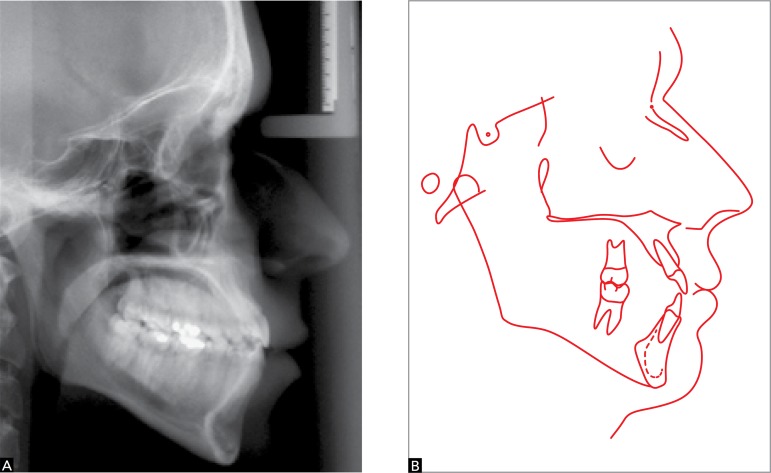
A) Final cephalometric profile radiograph and B) cephalometric tracing.
